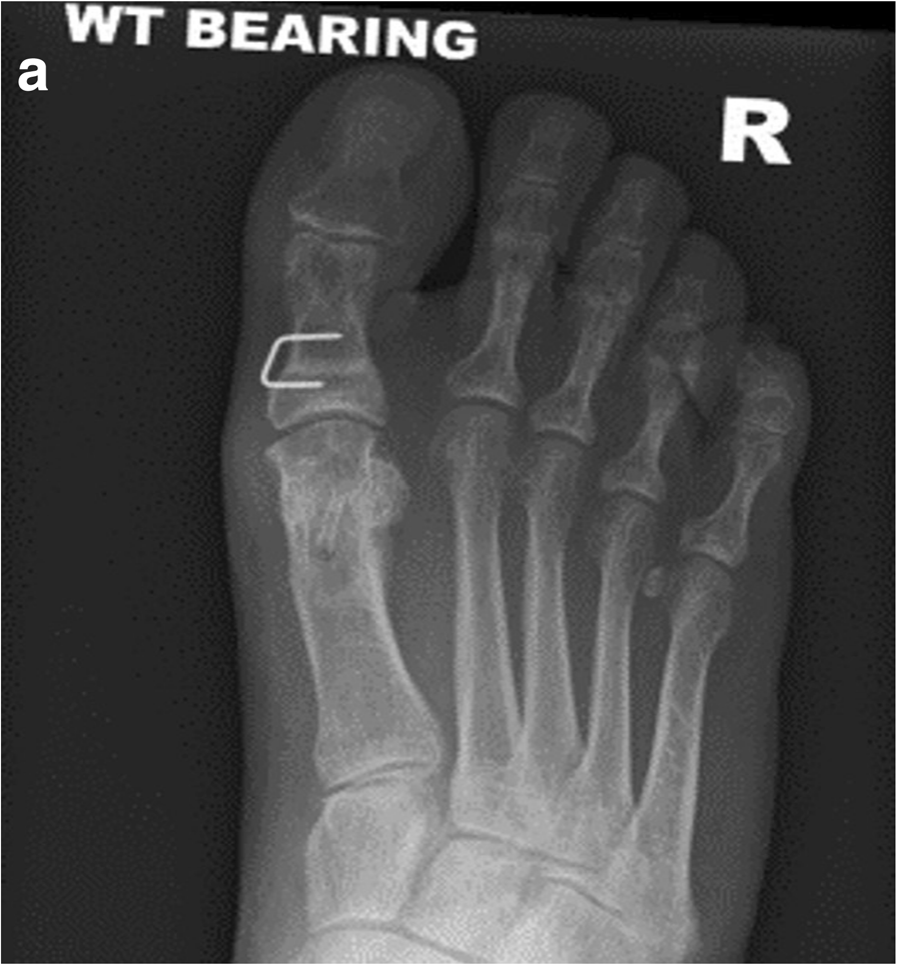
a and b Post-operative AP and lateral radiographs taken at 12 months follow up
